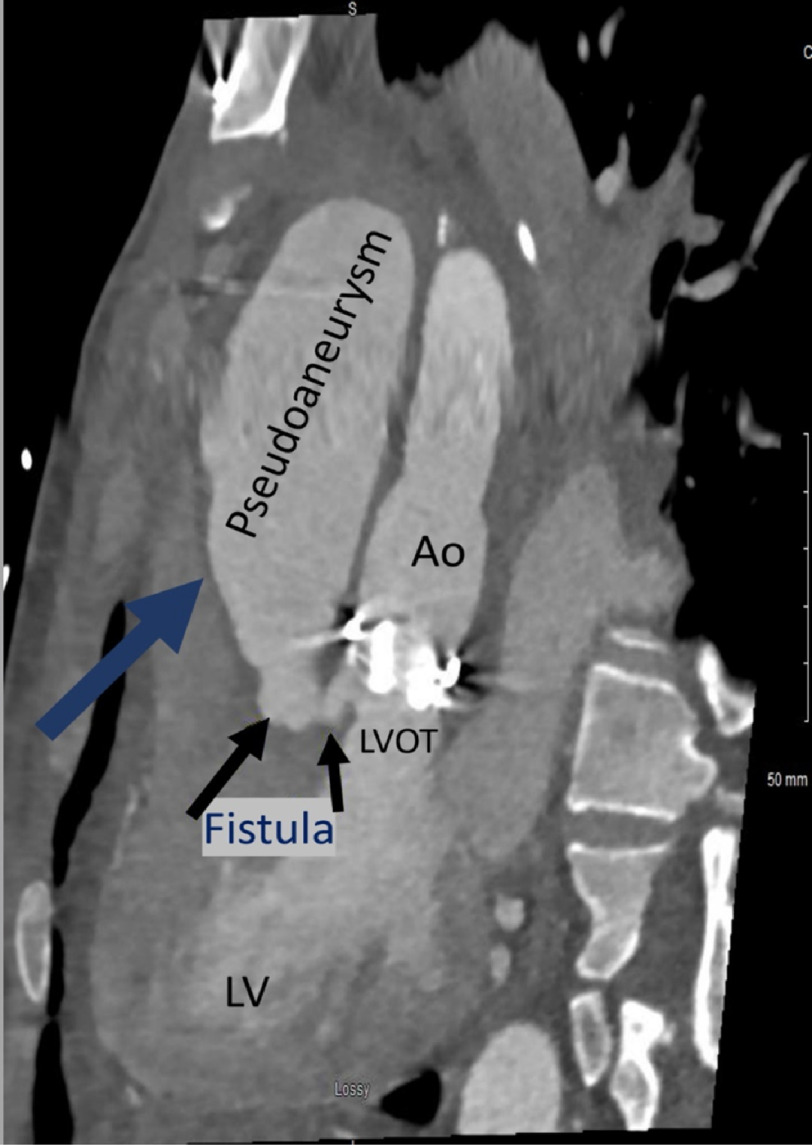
Dedicated coronary sagittal CT showing enlargement of the pseudoaneurysm with fistulous connection to LVOT.
